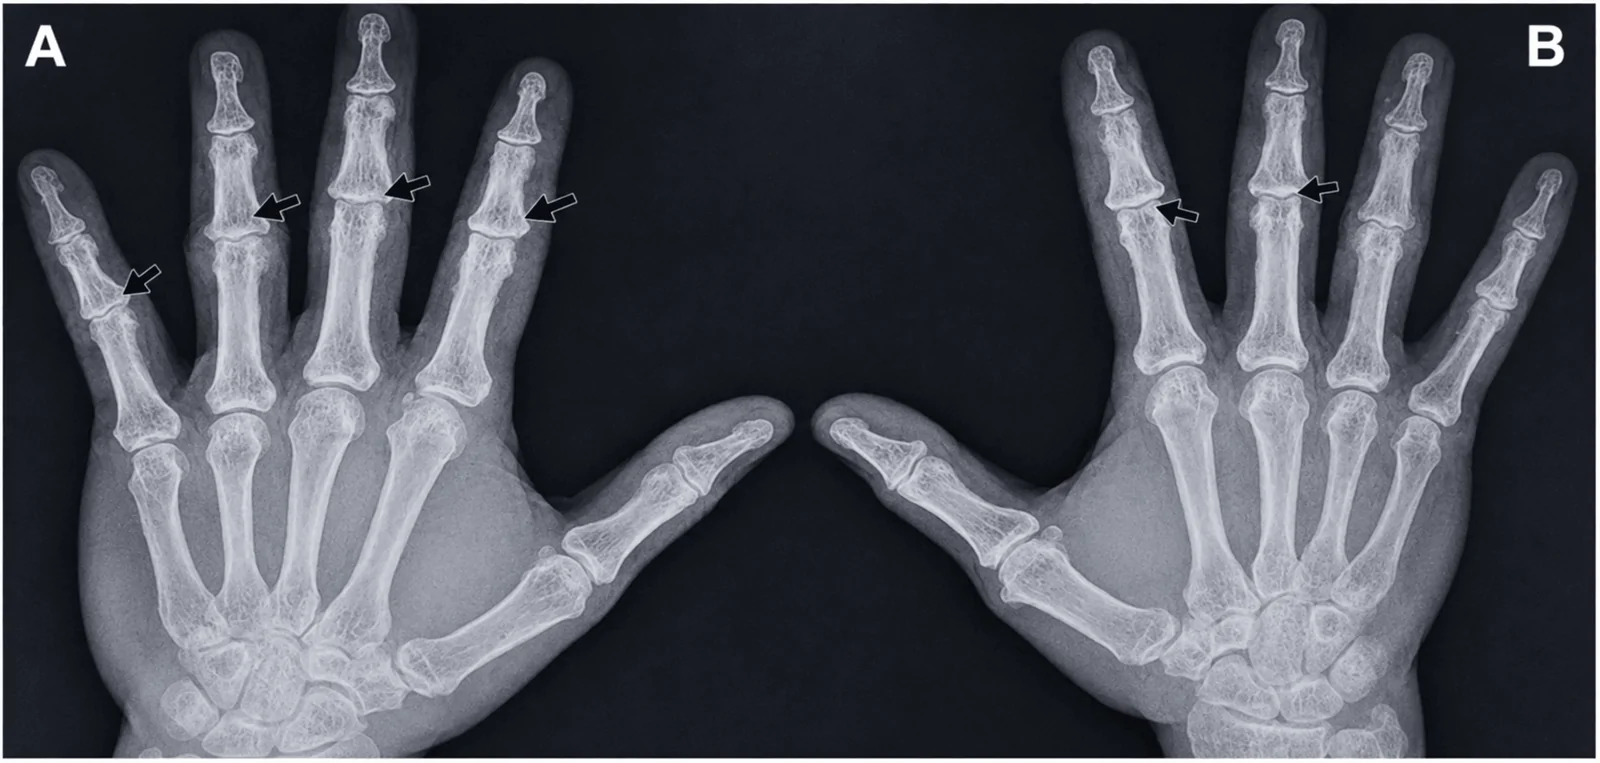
Bilateral hand radiographs demonstrating chronic gouty arthropathy. (A) Left hand showing multiple punched-out erosions with overhanging edges. (B) Right hand demonstrating similar erosive changes consistent with chronic gout.
